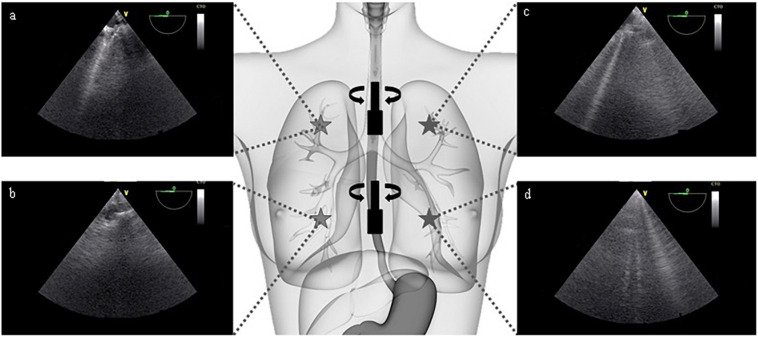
Selected transoesophageal views of the upper right (a), lower right (b), upper left (c), and lower left (d) lobes of the lung (patient #10). The transducer is introduced into the esophagus to a depth of 20–25 cm and then 30–40 cm below the incisors. At each step, the transducer is turned to the left and to the right, in order to avoid the heart and view the lung parenchyma. It should be noted that all images were captured at a multiplane angle of 0 degree. The view depth was set to 15 cm. Each image was captured during a 5-s end-expiratory occlusion.
